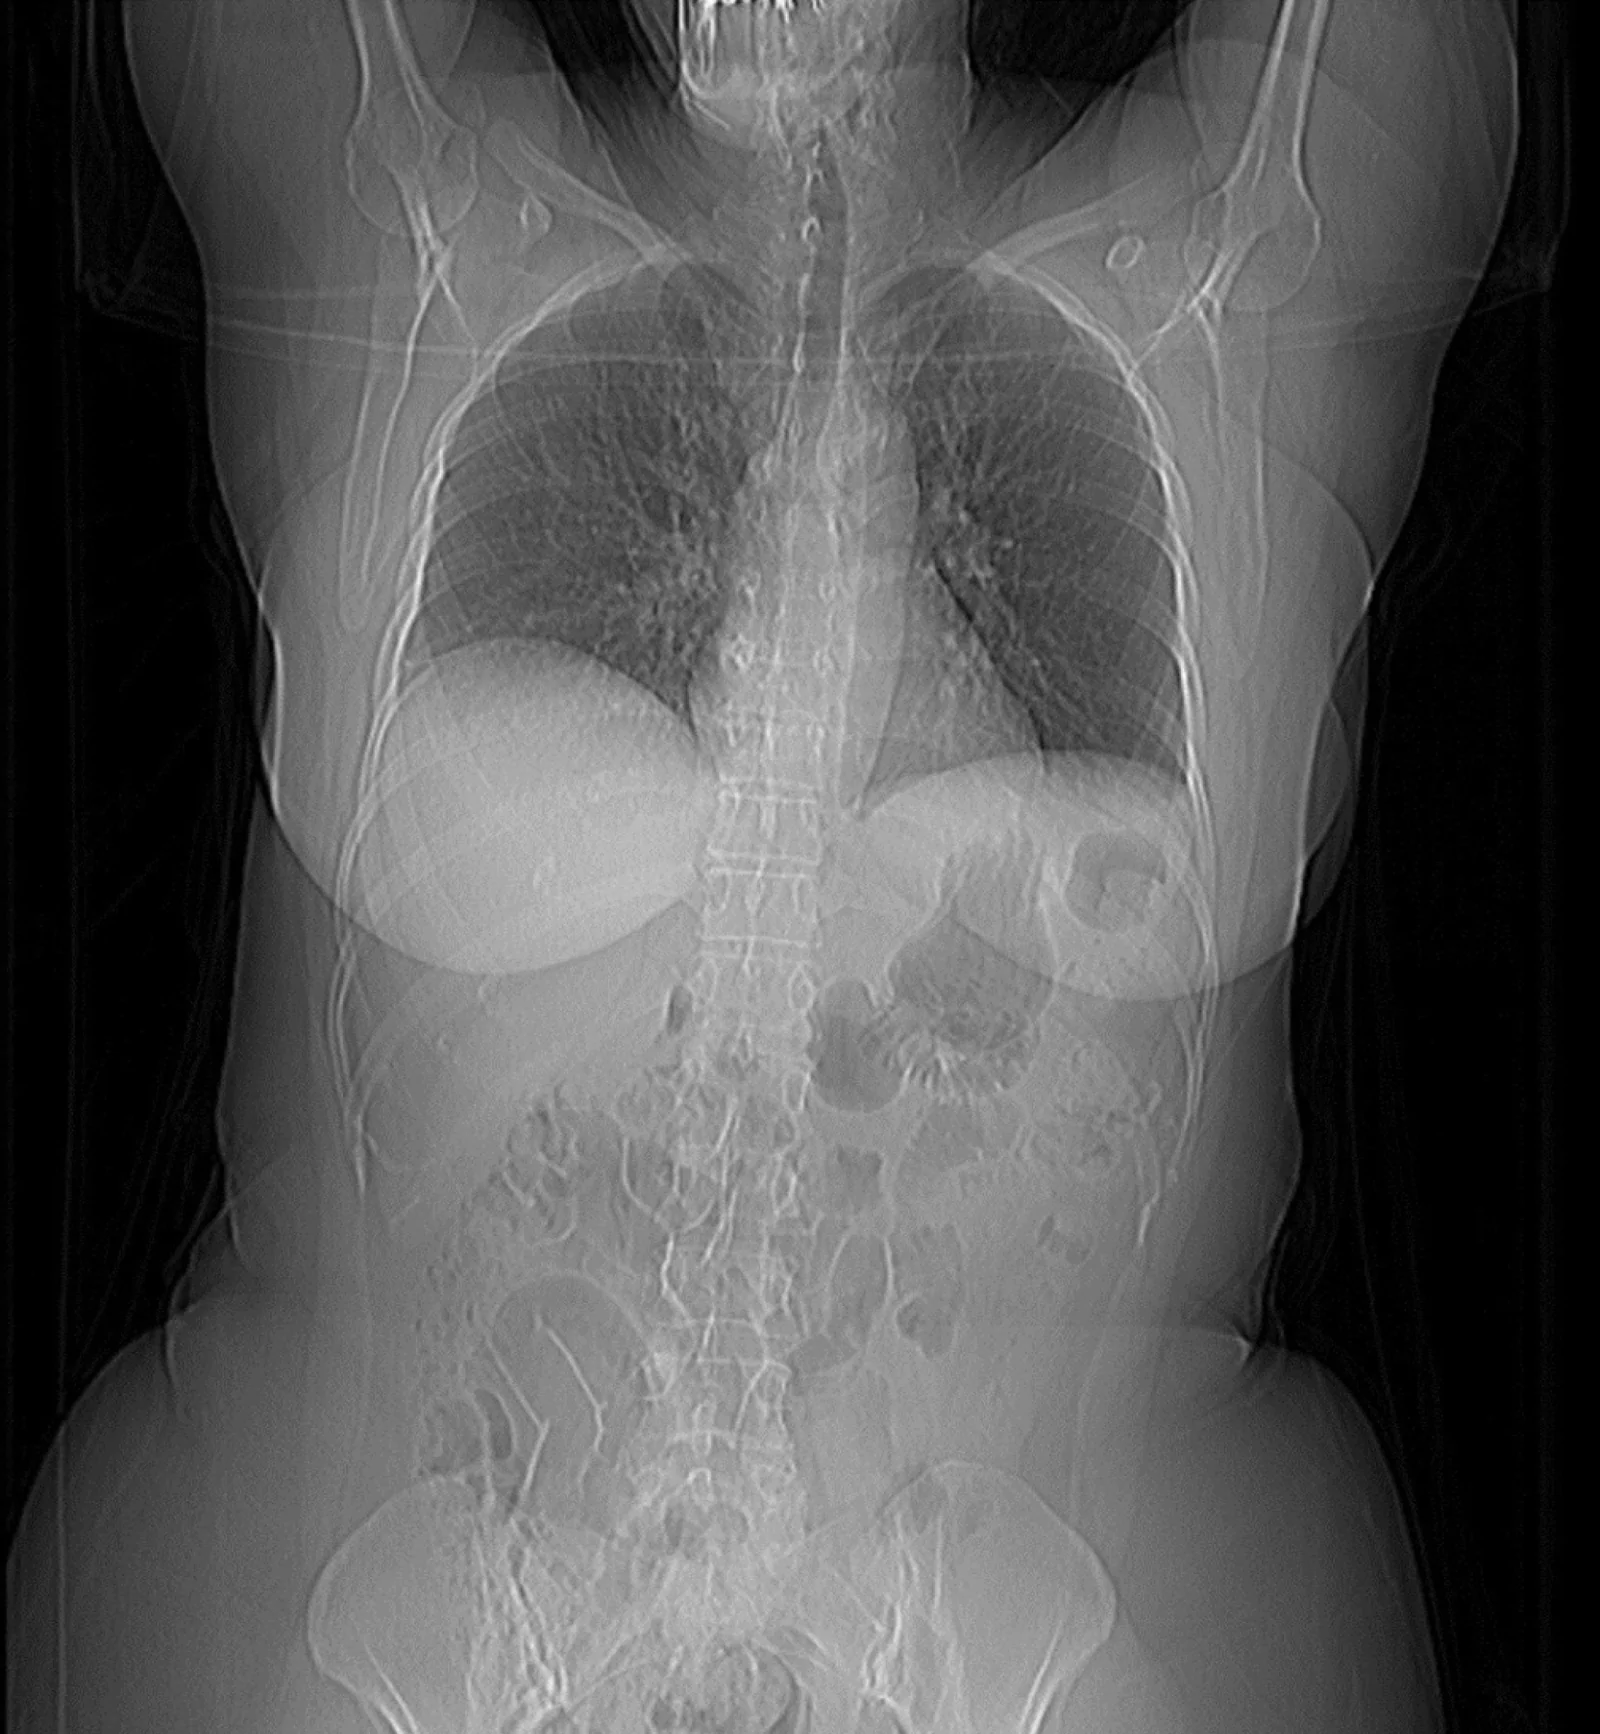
A scout thoracoabdominopelvic x-ray, failing to show any signs of pulmonary embolism or nephrolithiasis. Additionally, the breast shadows mask any potential pulmonary findings behind them.
